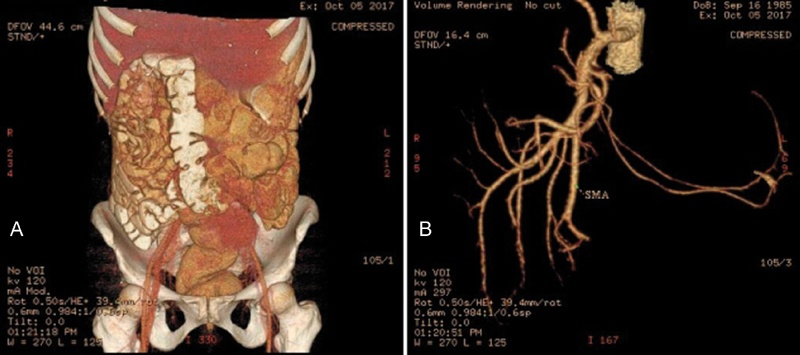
Computed tomography reconstruction images showing (
